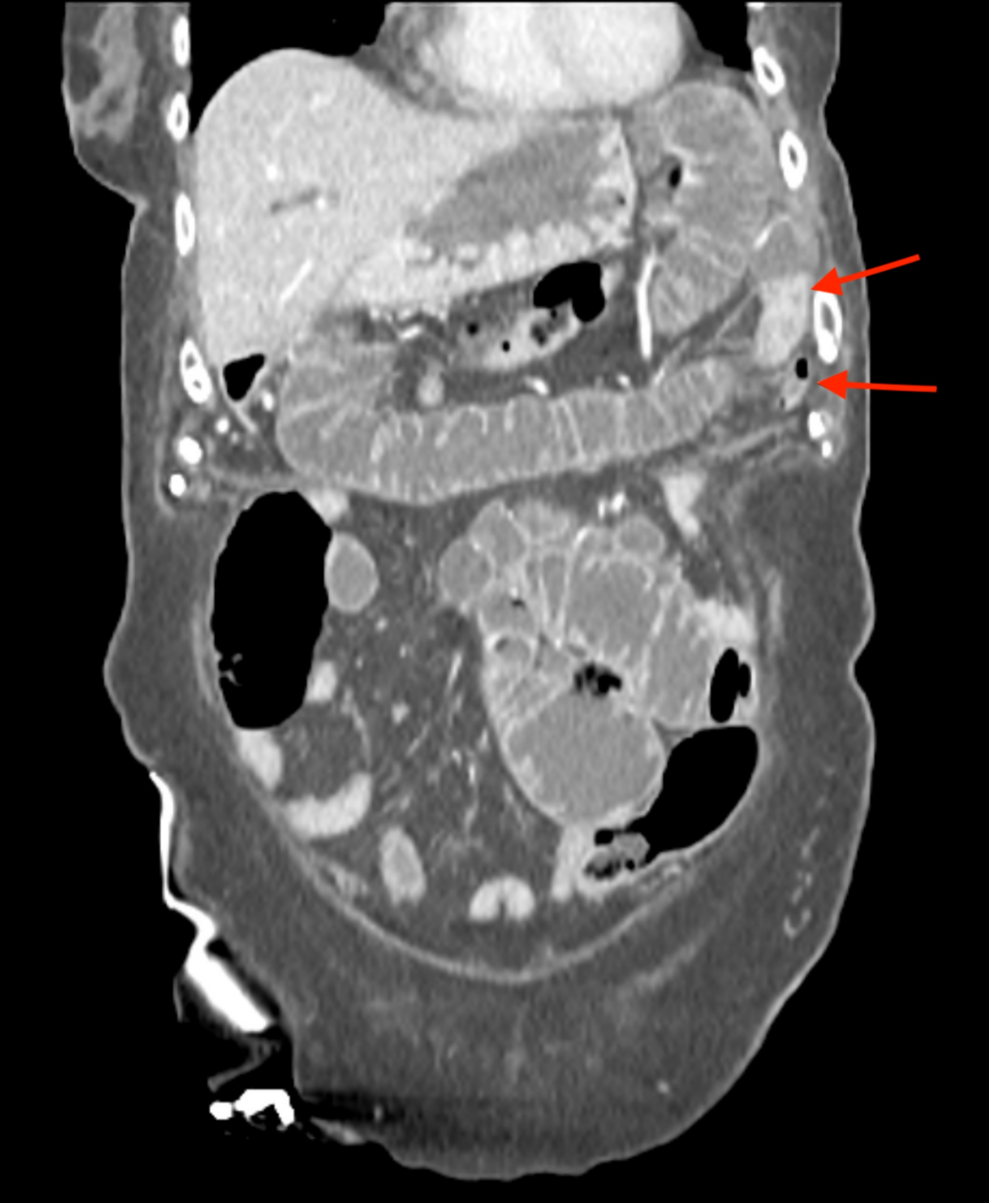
Coronal CT image showing the enterolith causing a high-grade mechanical bowel obstruction. This image demonstrates collapsed small bowel distal to the transition point (arrows).
